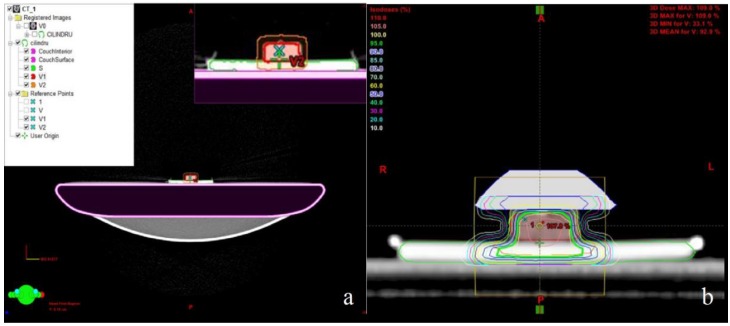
(a) The target volume delineation on the computed tomography (CT) images of the sample; (b) the dose distribution in volume of material after bolus application.
